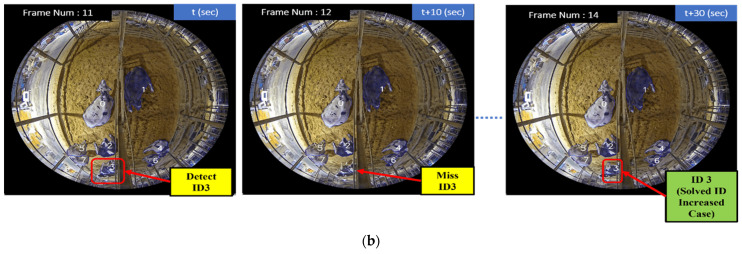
Comparison of miss detection case when using IOU and CTA: (a) IOU algorithm (which cannot solve the ID increment case); (b) CTA (which solved the ID increment case).
